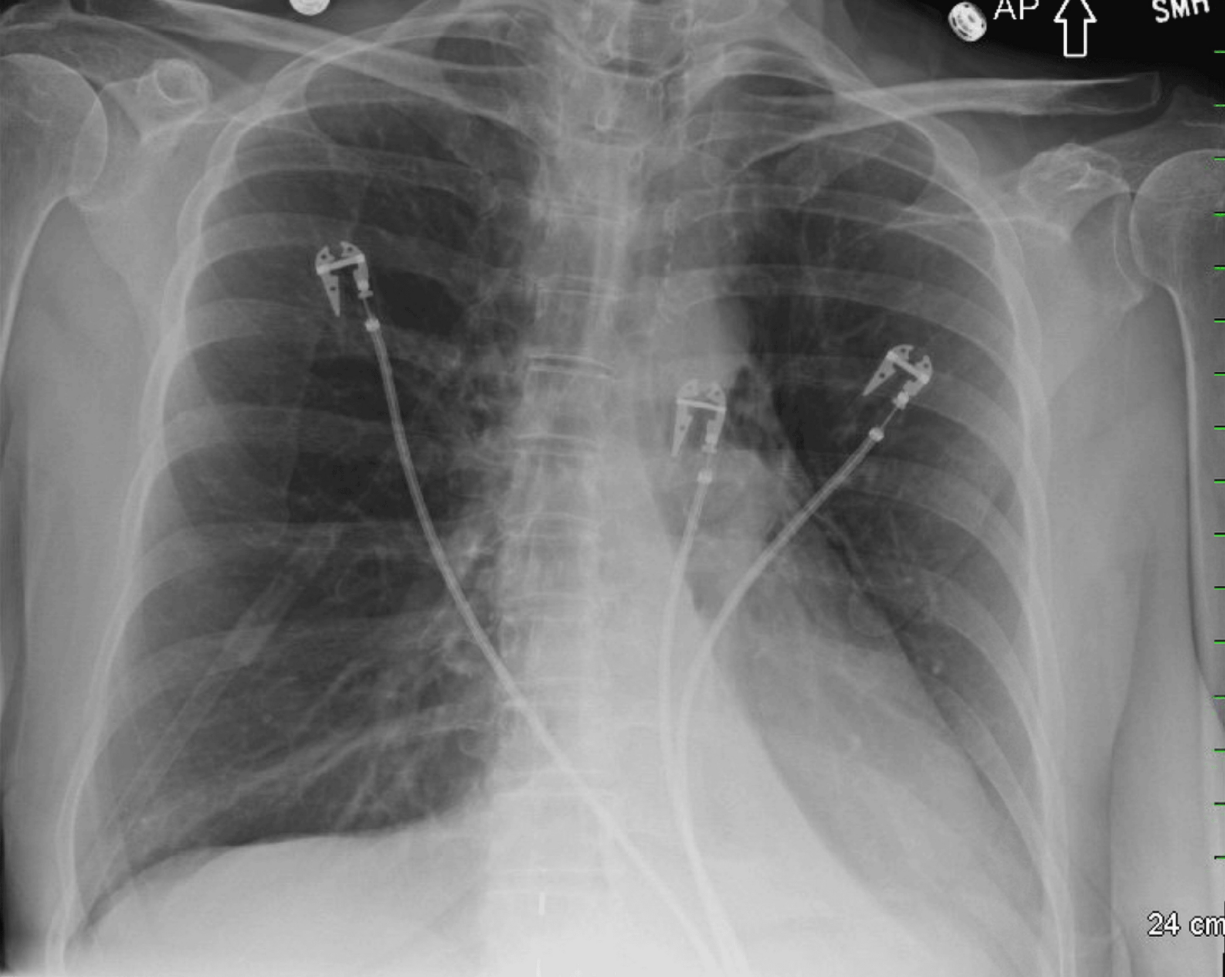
Portable chest radiograph on admission showing no evidence of PM PM, pneumomediastinum
